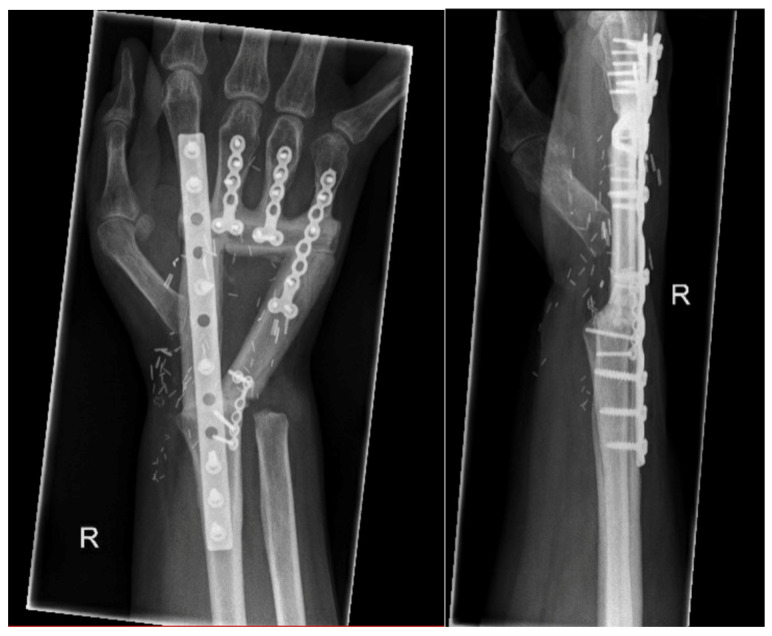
Reconstruction with a triangular shaped free osteocutaneous fibula flap and multiple fixation plates. Two years after the surgery, X-rays revealed a complete bone union.
